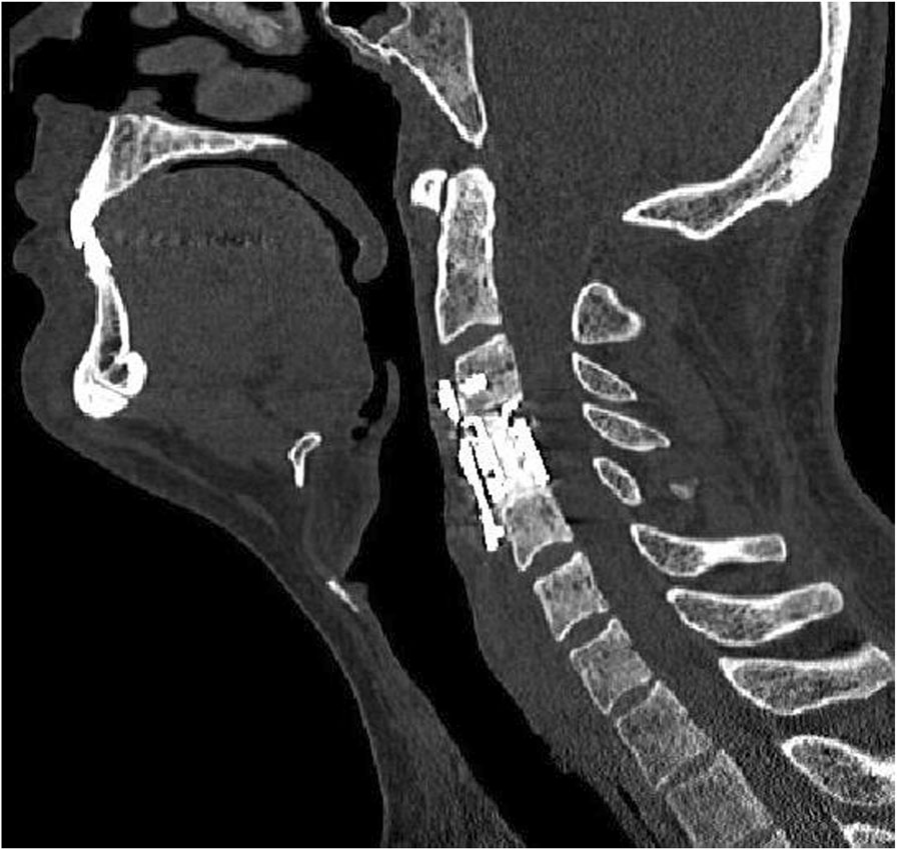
C4 corpectomy, corpectomy cage, and C3–5 anterior plate-screw image on postoperative CT in the sagittal plane
